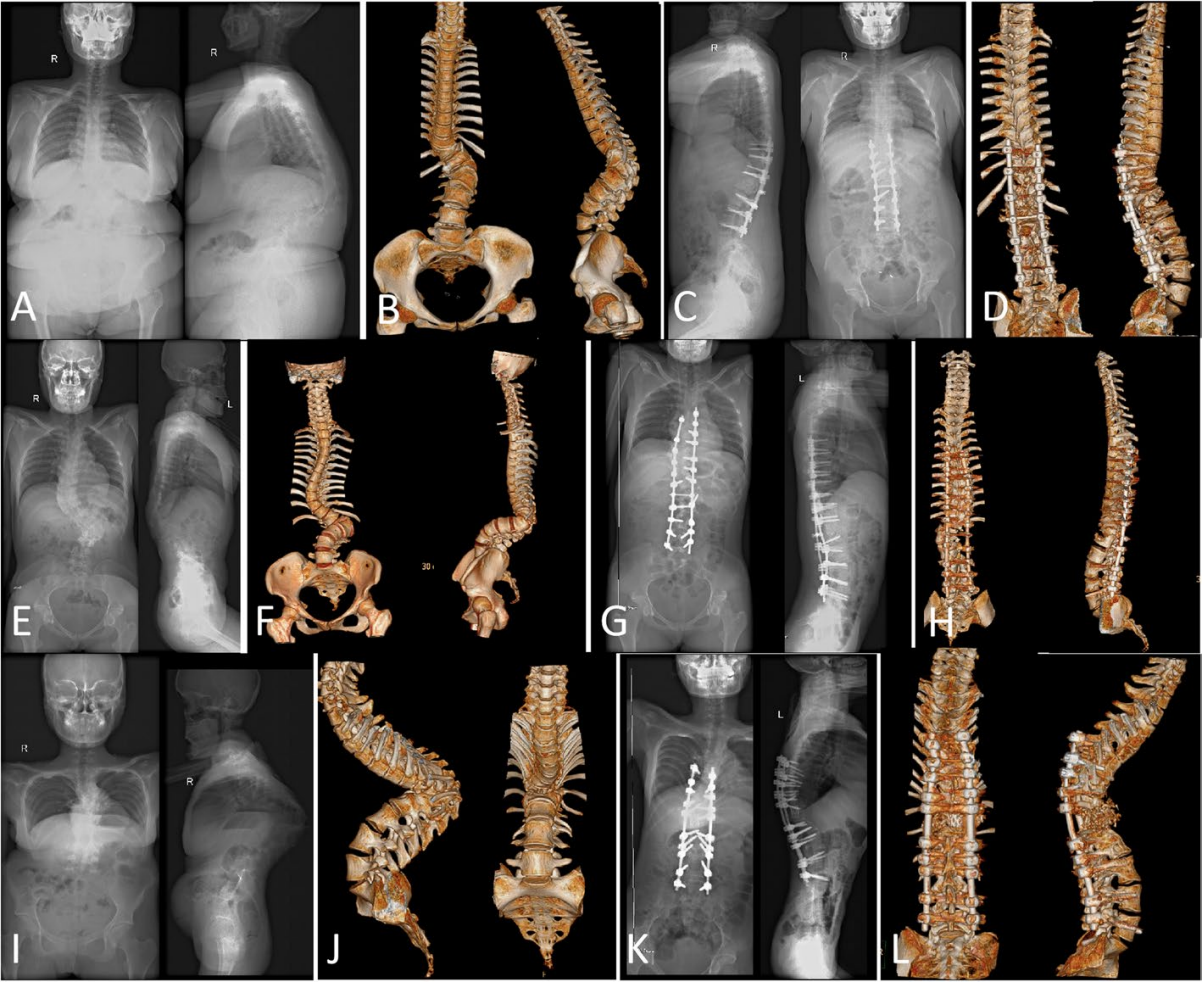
A 38-year-old woman with idiopathic kyphoscoliosis who received grade II PEO. A and B Preoperative reconstructed CT and radiographs showing scoliosis, Cobb (63°) and kyphosis, Cobb (85°). C and D Radiographs and reconstructed CT demonstrating the results of PEO with posterior spinal fusion; the scoliotic curve was corrected to 15° and the kyphotic curve to 25°. A 25-year-old woman with congenital kyphoscoliosis who underwent grade III PEO. E and F Preoperative reconstructed CT and radiographs showing scoliosis, Cobb (98°) and kyphosis, Cobb (112°). G and H Radiographs and reconstructed CT demonstrating the results of PEO with posterior spinal fusion: the scoliotic curve was corrected to 18° and the kyphotic curve to 20°. A 20-year-old woman with congenital kyphosis who received grade IV PEO. I and J Preoperative reconstructed CT and radiographs showing kyphosis Cobb, (166°). K and L Radiographs and reconstructed CT demonstrating the results of PEO with posterior spinal fusion and the titanium mesh implants for the front support of the vertebra; the kyphotic curve was corrected to 46°
